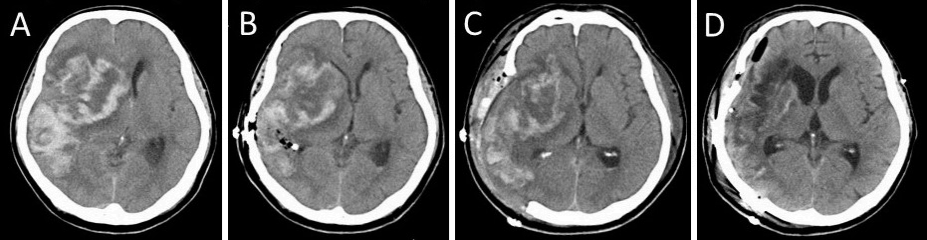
Head computed tomography (CT) indicated hemorrhagic infarction within a known cerebral infarct, midline shift, and cerebral herniation (A). Postoperative head CT indicated a progressively less midline shift after small craniotomy (B), right frontotemporal large decompressive craniectomy (C), and cranioplasty (D).
